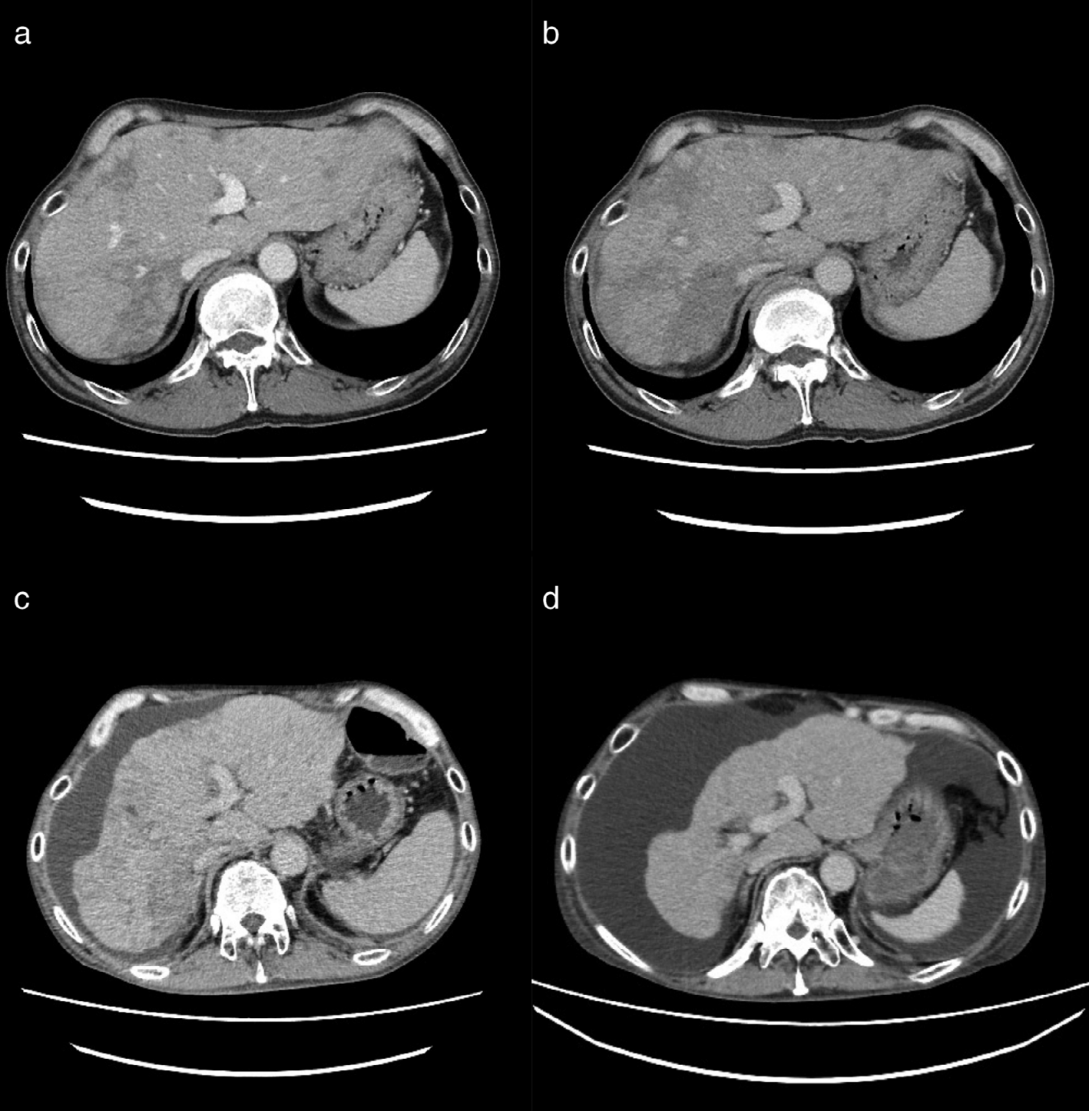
Computed tomography (CT) findings of liver metastasis and liver morphology over time. (a) Three months after the first visit, a decrease in the low‐density area in the tumor was noted in response to first‐line treatment. (b) Five months after the first visit, during maintenance therapy, an increase in the low‐density area was observed, and second‐line therapy was initiated. (c) Seven months after the first visit, the patient responded to second‐line therapy, and a decrease in the low‐density area of the tumor was observed; however, ascites appeared, followed by liver shrinkage. (d) Nine months after the first visit, a further increase was observed in ascites, the liver showed cirrhosis‐like morphology, and the patient's performance status worsened
